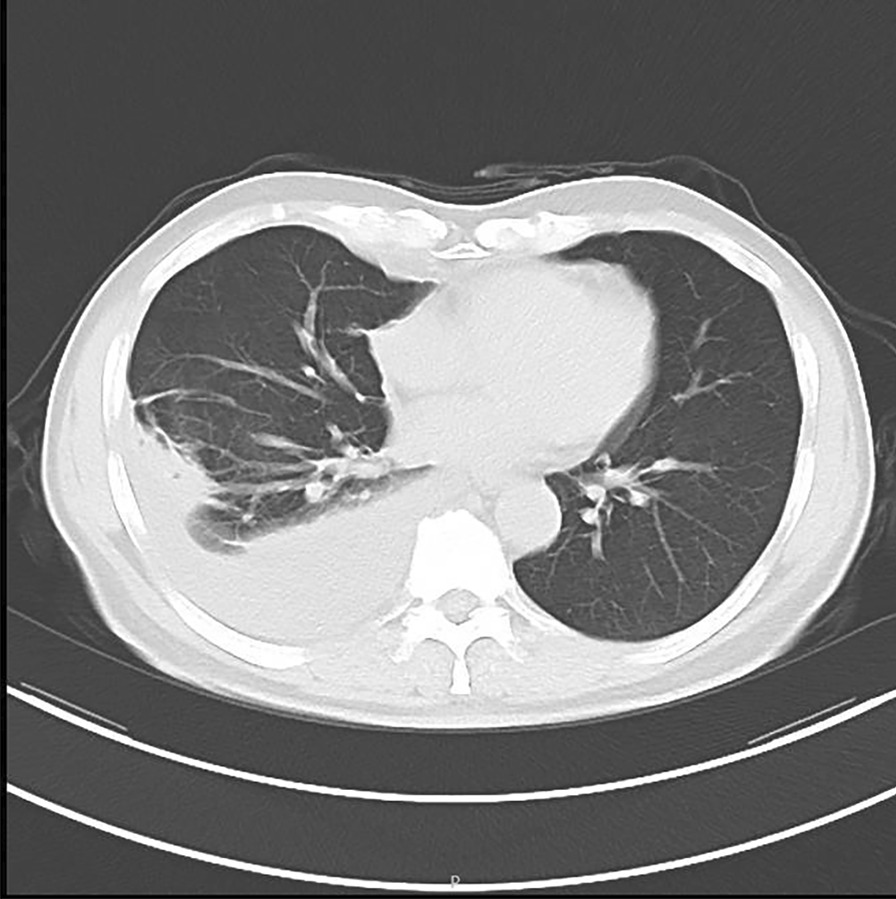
CT of the lung: patchy shadow can be seen in the lower lobe of the right lung, and effusion signs can be seen in the right chest cavity with hypodynamia of the lower lobe of the right lung
